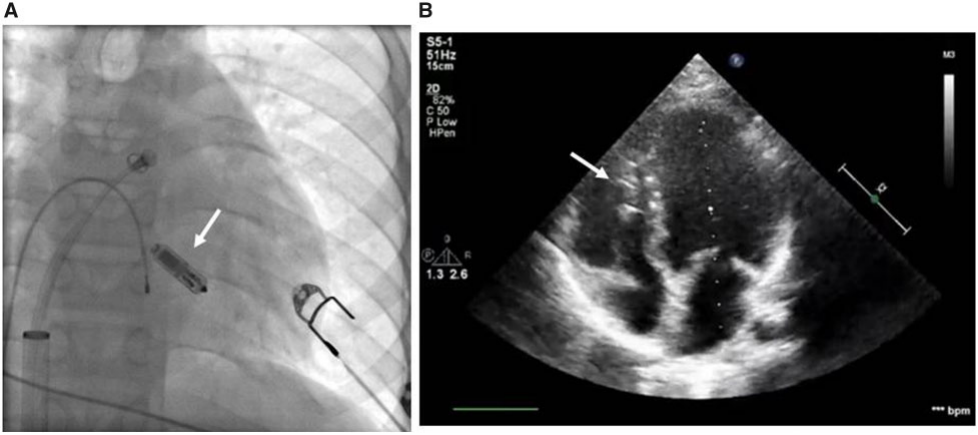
Location of the Micra™ device in fluoroscopy during the implantation procedure (*A*) and in transthoracic echocardiography (*B*) in a Patient 1. Location is marked with white arrows.
